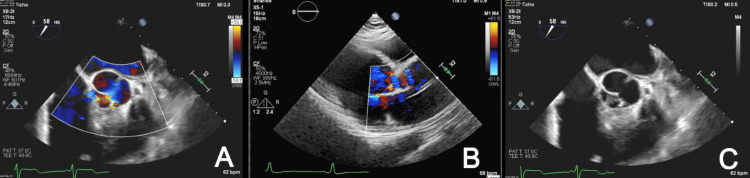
Transesophageal echocardiogram demonstrating aortic regurgitation during diastole in the parasternal short axis on the Doppler (A), aortic regurgitation in the parasternal long axis with the Doppler (B), and vegetation on the aortic valve (C).
